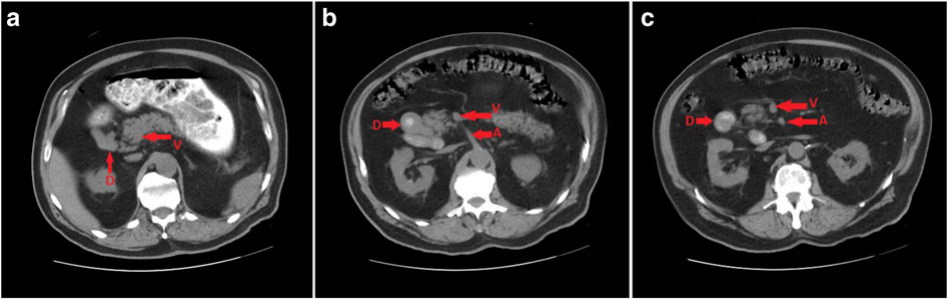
A CT scan without IV contrast showed generalized esophageal wall thickening and mild duodenal (marked by “D”) wall thickening (b). The duodenal sweep does not cross the midline (a–c), consistent with a malrotation. There is no obstruction identified. The transverse colon evaluation was limited due to motion blur. The SMV (marked by “V”) is anterior and to the right of the SMA (marked by “A”), as would be found normally. EUS performed showed a duodenal mass in the second portion of the duodenum that measured 3–4 cm. CT, computed tomography; EUS, endoscopic ultrasound; SMA, superior mesenteric artery; SMV, superior mesenteric vein.
